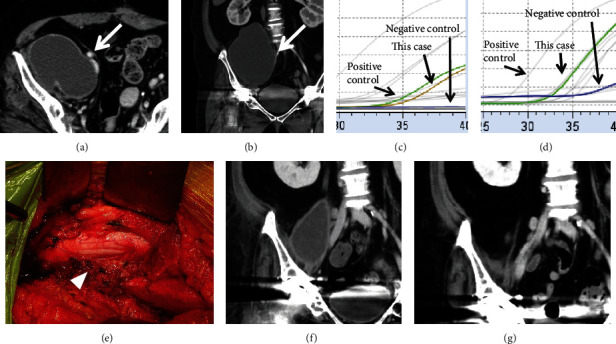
X-ray and computed tomography (CT) images at first presentation. Axial image (a) and coronal image (b) of the CT at first presentation show a mass region with a major axis of 10 cm in accordance with the right iliac and psoas muscles that compress the right artery and vein (white arrow). No mass is found around the hip joint. MRS-PCR shows positive for *mecA* gene detection (c) and 16S rRNA gene detection (d) despite a culture-negative result. Intraoperative finding shows the mass region's extensive amount of fluid that compresses the femoral nerve from the retroperitoneum side (e). CT showed residual mass region 2 weeks after surgery (f), which disappeared three months after surgery (g).
